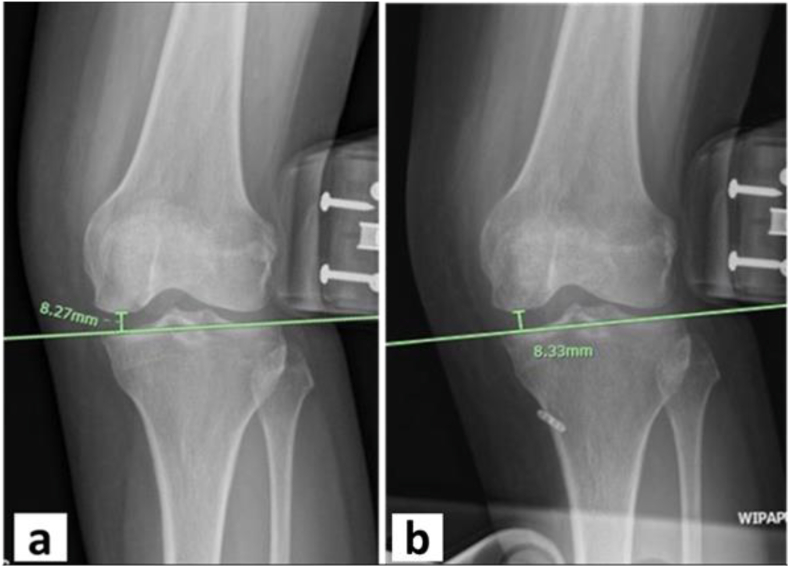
(a) Showing the radiographic valgus stress of left knee (AP view) in a patient with a medial meniscus root tear. The preoperative medial joint space width was 8.27 mm. (b) Showing the radiographic valgus stress of left knee (AP view) in a patient underwent medial meniscus root repair using the transtibial single tunnel pullout technique at 3 months postoperatively. The medial joint space width was 8.33 mm at 3 months postoperatively.
